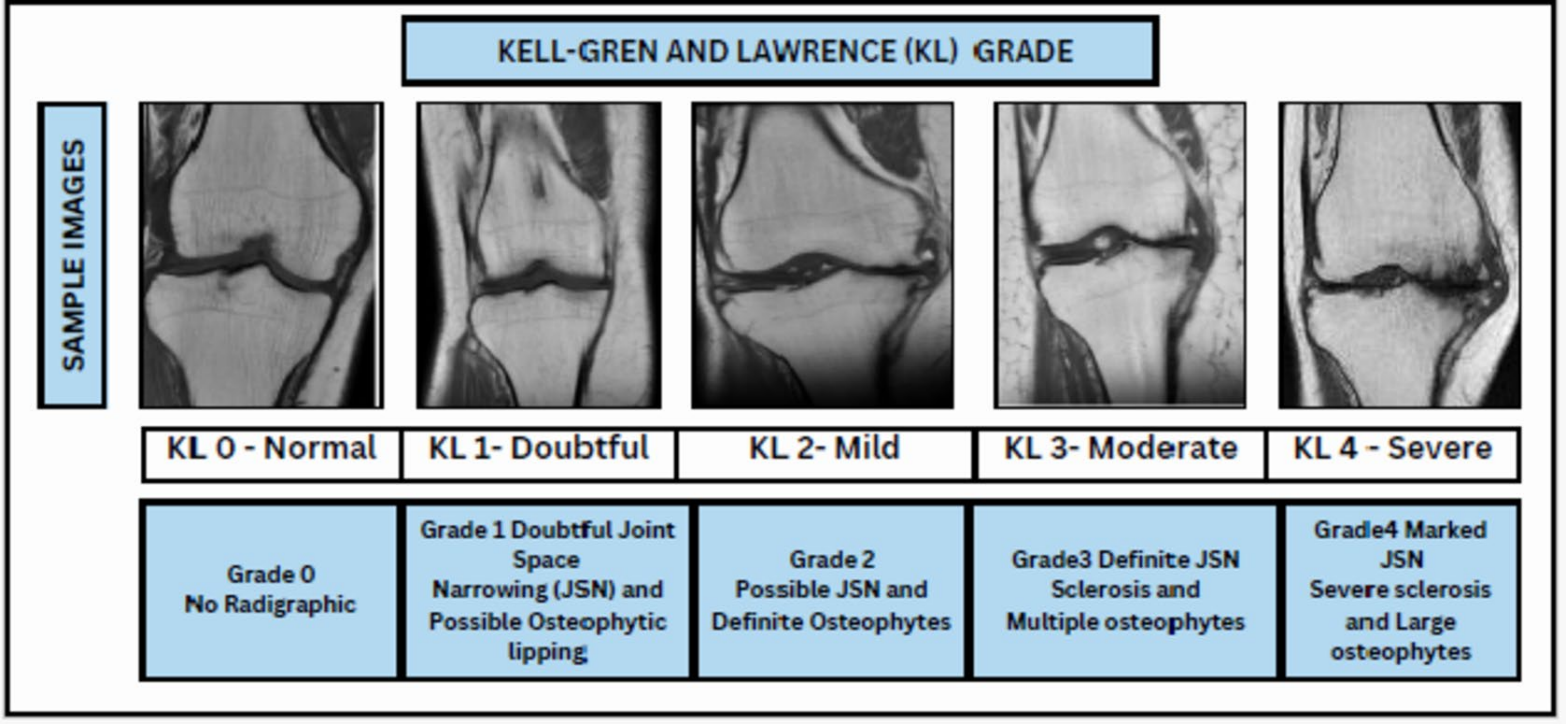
KL- grades distribution of Right Knee MRI.
